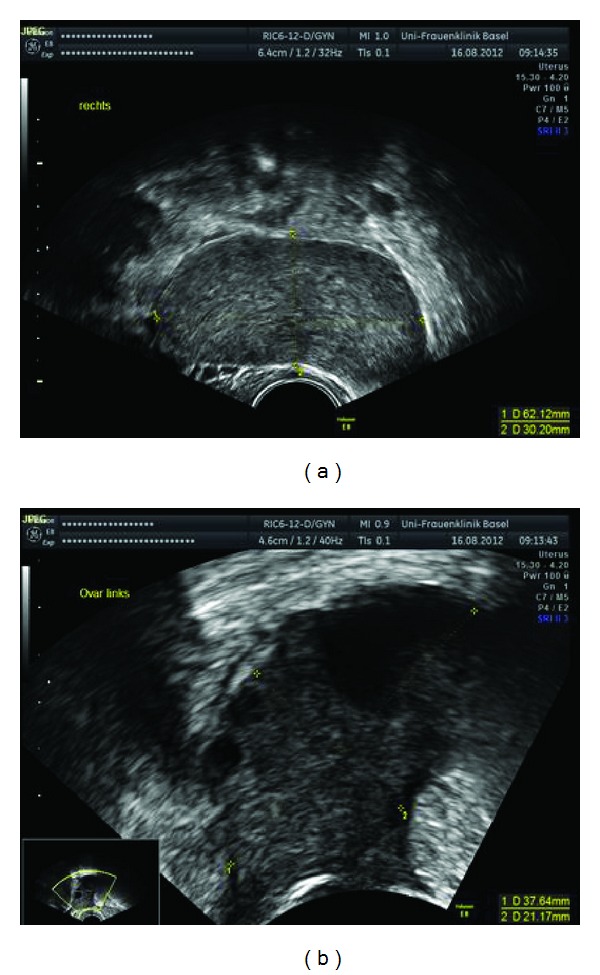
Ovarian ultrasound after 1st cycle of chemotherapy.
